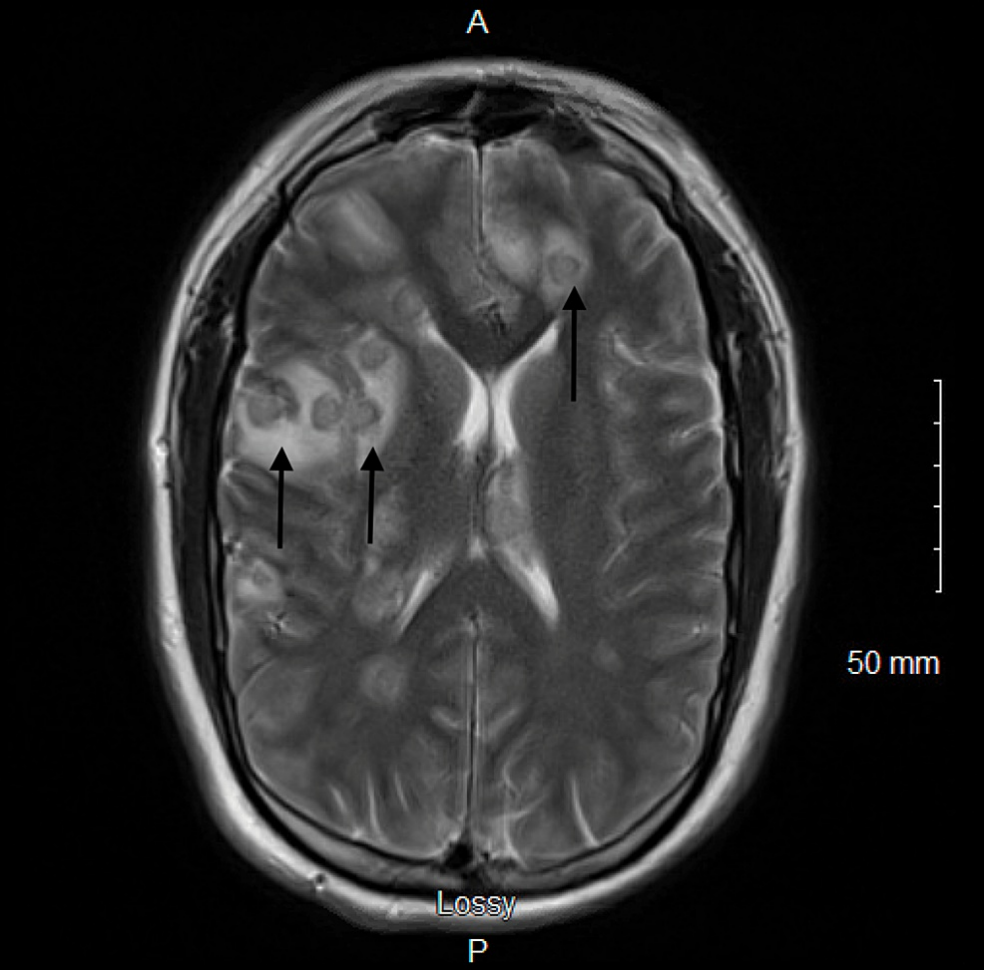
T2-weighted magnetic resonance image sequence of the brain demonstrating multifocal foci with hypointense thin rims around the abscesses and surrounding high-signal-intensity edema.
